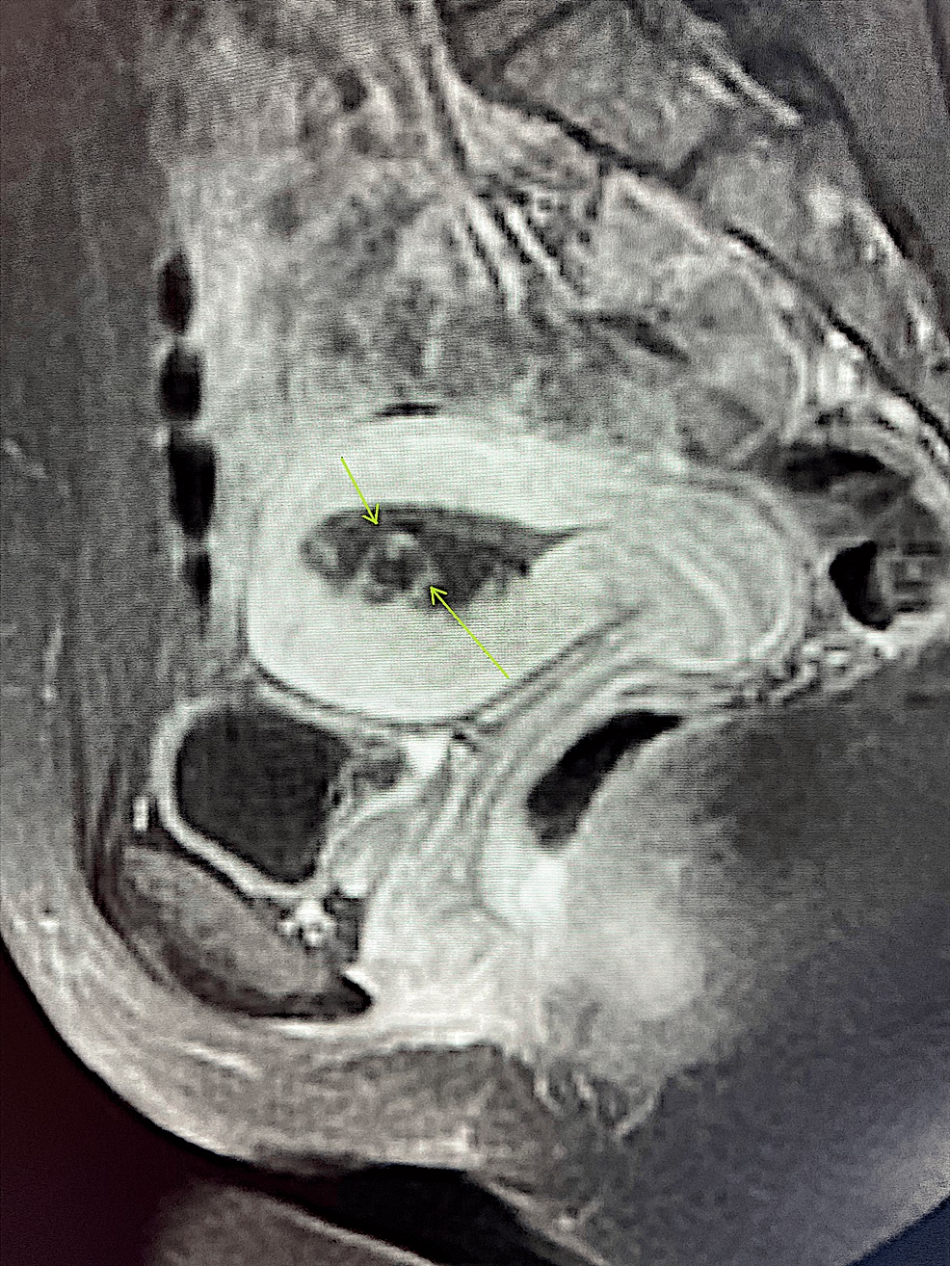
Sagittal T1 post-contrast MRI. Arrows point towards abnormal enhancement in the endometrium.
